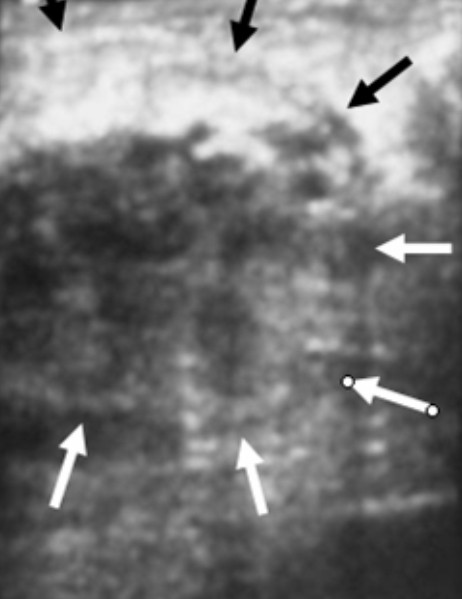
An ill-defined, hypoechoic, heterogenous 6 cm lesion is seen in the upper-inner quadrant of the left breast on ultrasonography.
